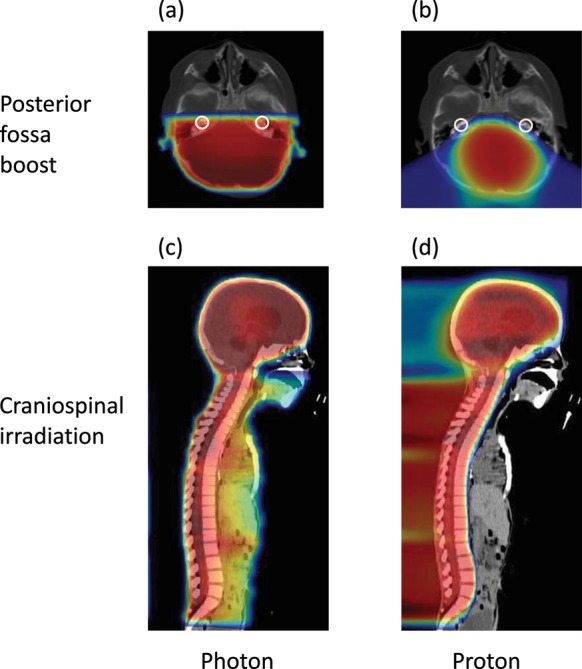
Treatment planning data of a representative case with medulloblastoma. Dose distribution in the transaxial plane of the posterior fossa for boost irradiation to the tumor bed with X-ray (a) and proton (b) beams. Dose distribution of sagittal plane for craniospinal irradiation with X-ray (c) and proton (d) beams. The cochleae are indicaed by white circles.
